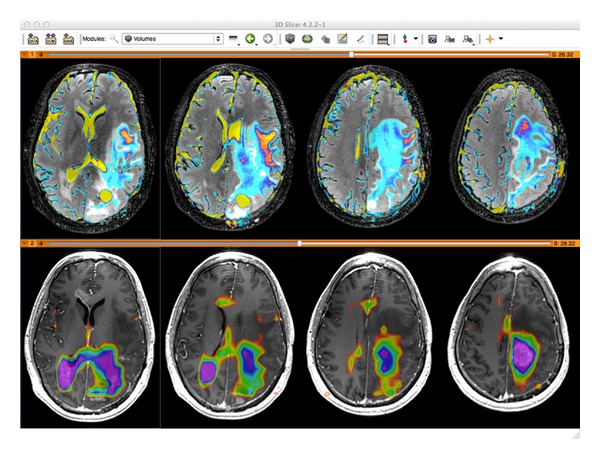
CNI metabolite maps (bottom color overlay) derived from MRSI data in SIVIC are exported as standard DICOM MR Image Storage SOP instances, which can be loaded into 3D DICOM image analysis software packages (shown here in 3D Slicer). Derived maps are thus amenable to multimodal analysis. The top panel shows ADC maps (color) on FLAIR images. The bottom panel shows the same anatomical locations on a T1 contrast enhanced image.
